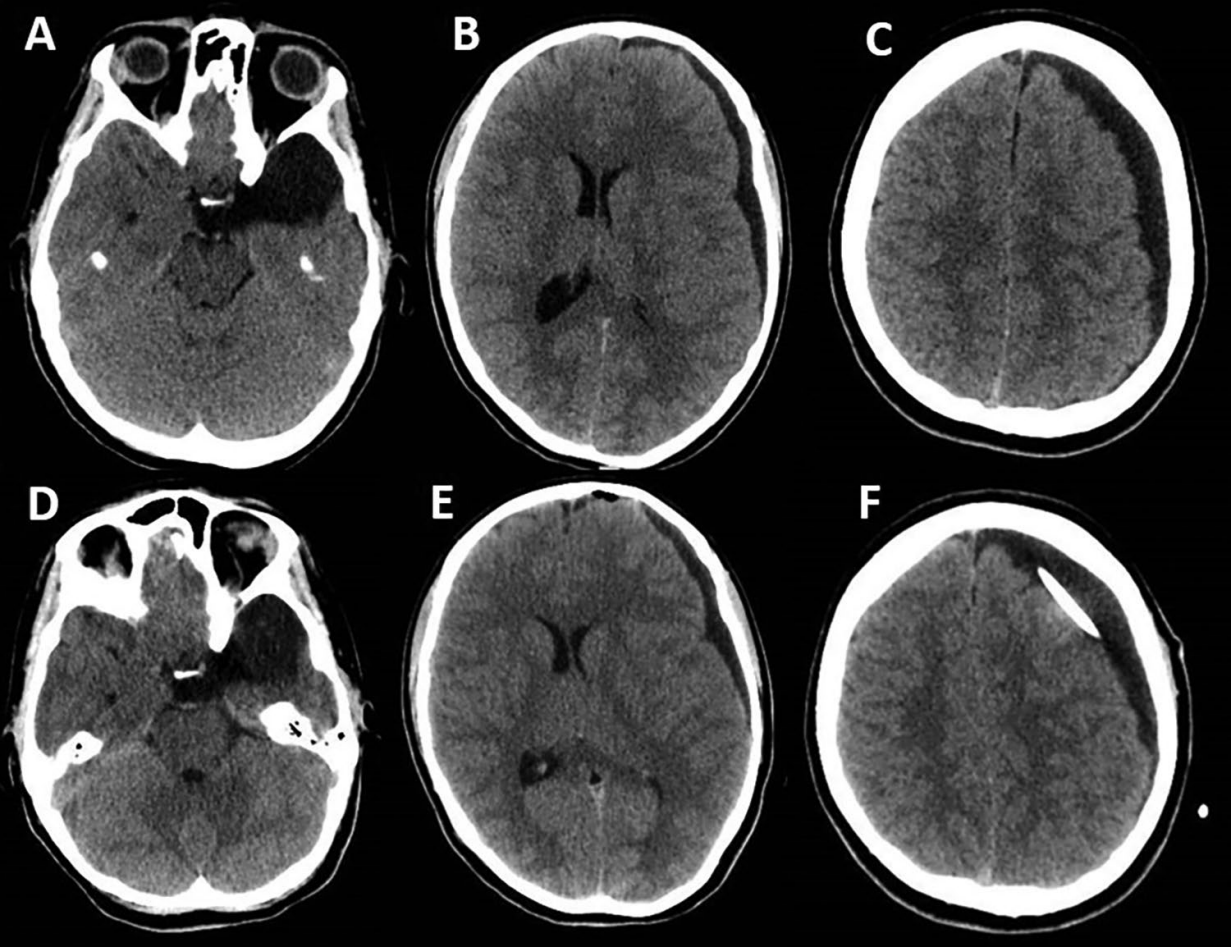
A–C CT scan showing the increase of the hygroma; D–F CT scan performed a few days after the placement of a subduro-peritoneal shunt, with no significant changes compared with the previous one
